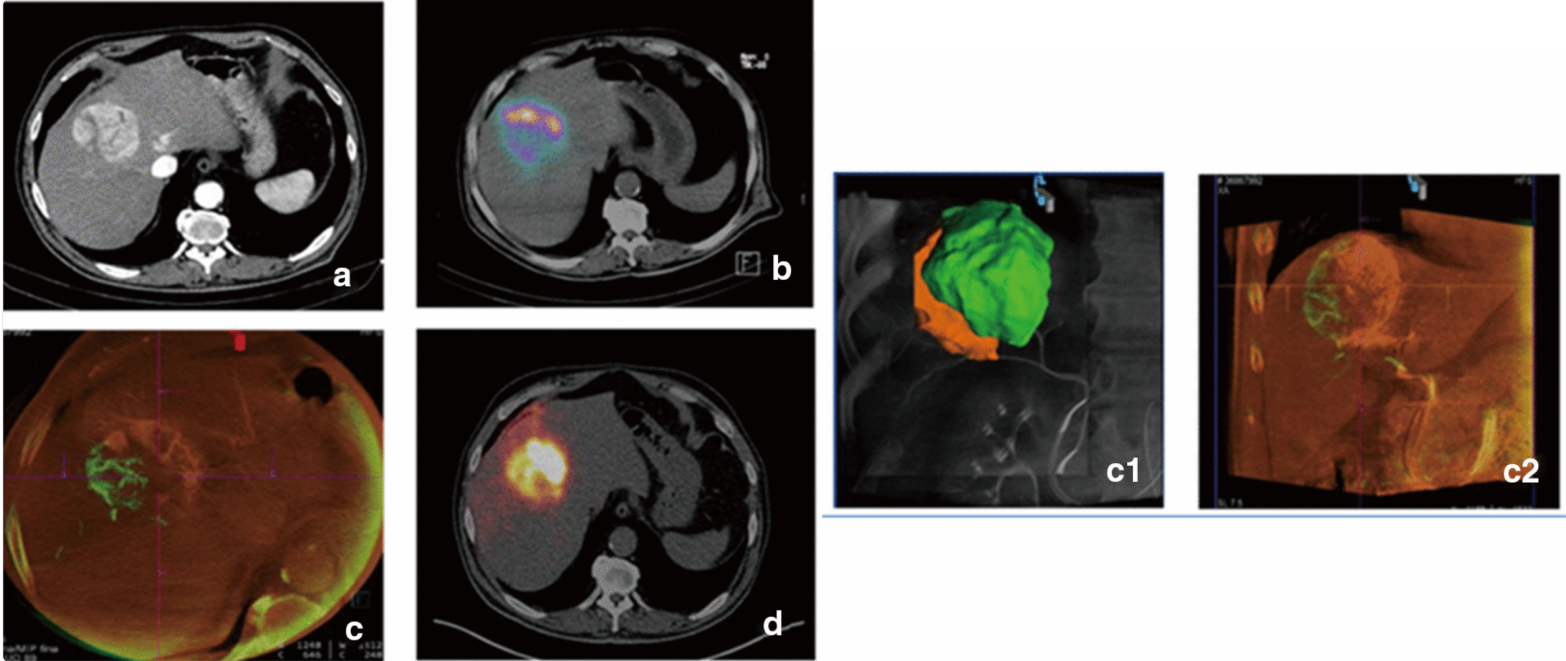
(a) Contrast-enhanced computed tomography (CECT) image: HCC located between segments IV and VIII. (b)^99m^Tc-MAA SPECT/CT fusion image shows low uptake in the lateral part of the tumoral nodule. ^99m^Tc-MAA activity was split in two doses of 50% each by IR decision, based on liver and tumoral volumes. (c, c1 and c2) C-arm cone-Beam CT (CBCT) volumetric assessment of the tumoral territory perfused by each artery. VIII segments artery (in green) fed only 32% of the tumoral volume while IV segment artery fed most of it (in orange). (d)^90^Y PET/CT fusion image after splitting the activity according to CBCT volumes shows the adequate distribution of the microspheres throughout the lesion (80). Reproduced from Rodríguez−Fraile et al., EJNMMI Research, 2021, CC BY 4.0.
